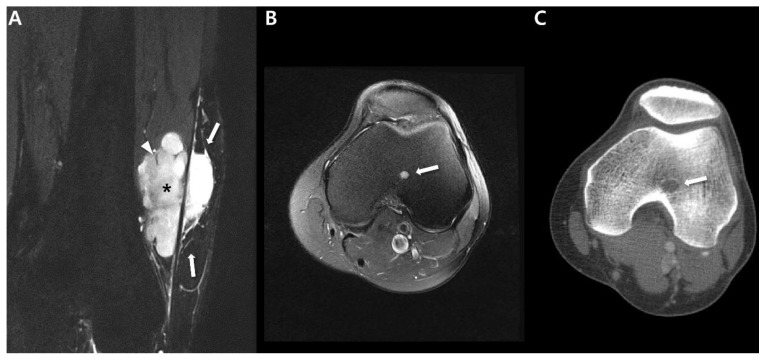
Bone metastasis from synovial sarcoma (same patient with Figure 8). (A) Initial MRI of coronal T2-weighed, fat-suppressed show about 5.2 cm sized soft-tissue mass (*) with intervening septa (arrowhead) showing ‘bowl of grapes’ and intercompartmental extensions (arrows). (B) Follow-up MRI of axial T2-weighted, fat-suppressed image after 3 years shows small round bone metastasis (arrow). (C) Metastatic lesion shows osteolytic appearance (arrow) on CT.
